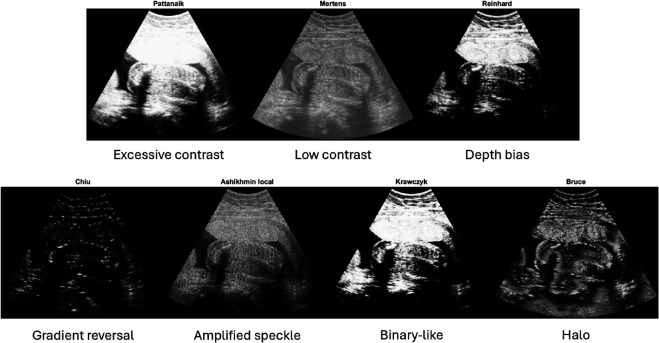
Examples of common failure modes observed in tone-mapped ultrasound images. Row 1:
